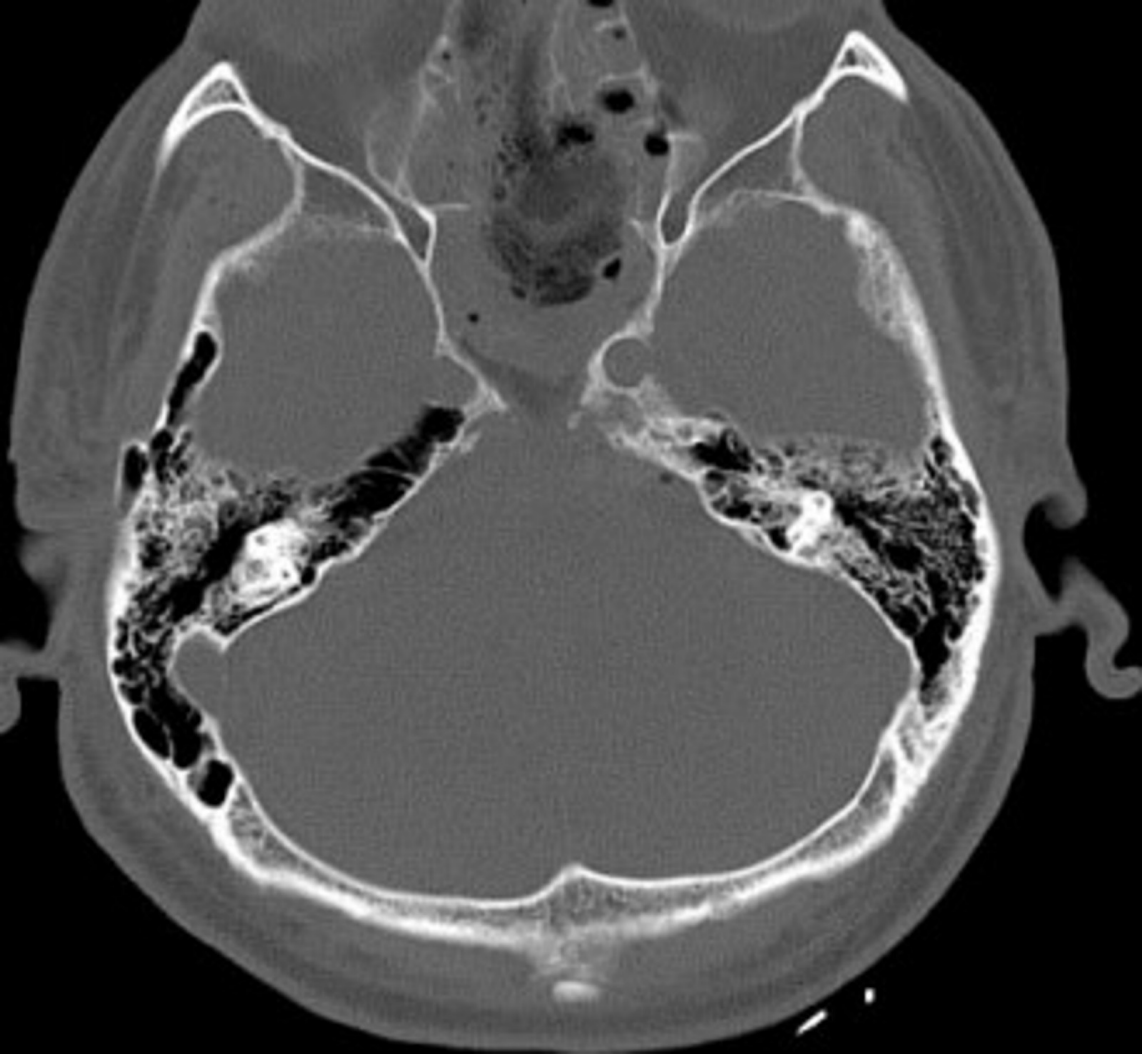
Postoperative CT Imaging Postoperative axial CT showing the extent of the bony clivus resection.
